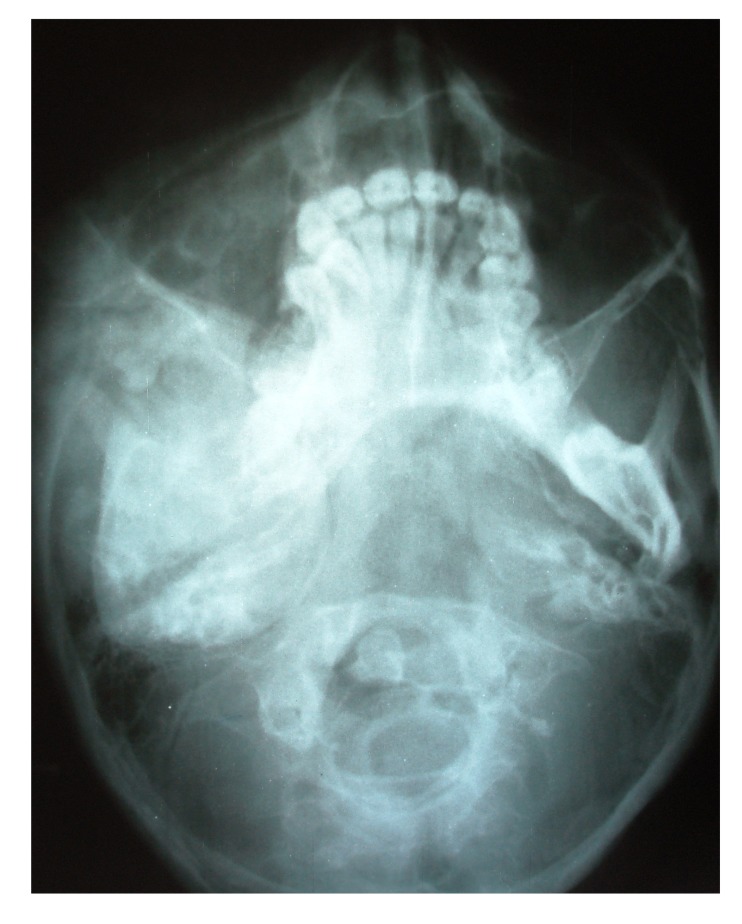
Submentovertex radiograph revealing expansion of the mandible of left side.
